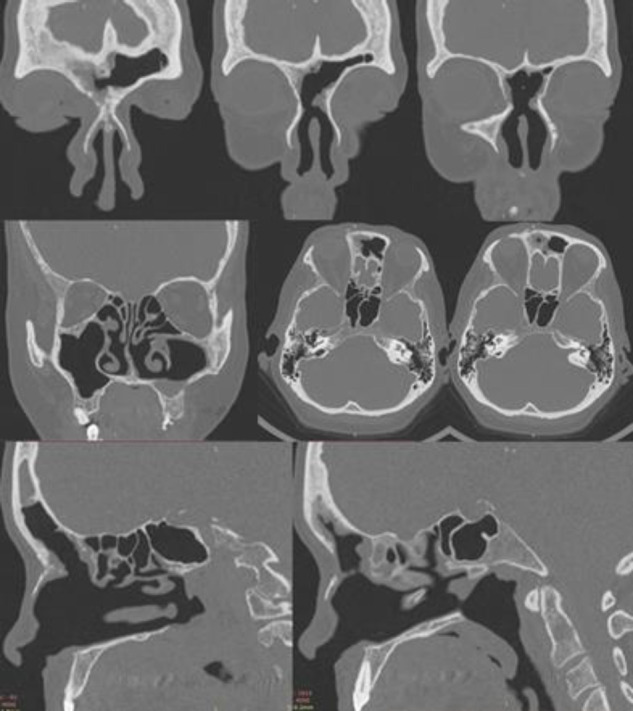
Paranasal sinuses two-month CT scan after operation (Modified lothrop procedure condition): free frontoethmoidal area with complete bilateral drainage, free inferior maxillary antrostomy with complete inferior meatus drainage
